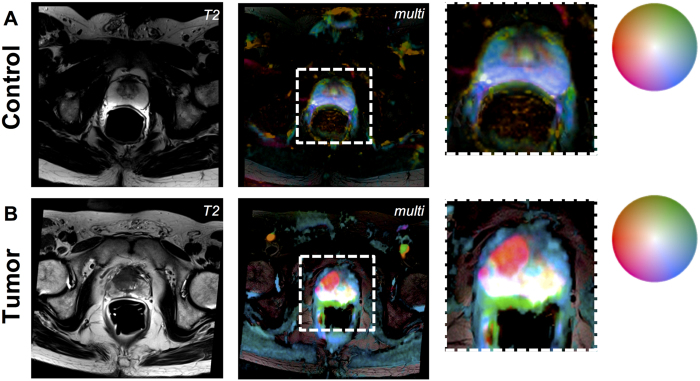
Examples of a control prostate and prostate cancer. (A) Patient with no evidence of prostate cancer (left: T2-weighted image, middle: multi-colored visualization of perfusion and diffusion imaging, right: enlarged detail). (B) Prostate cancer patient, panel layout corresponding to A.
